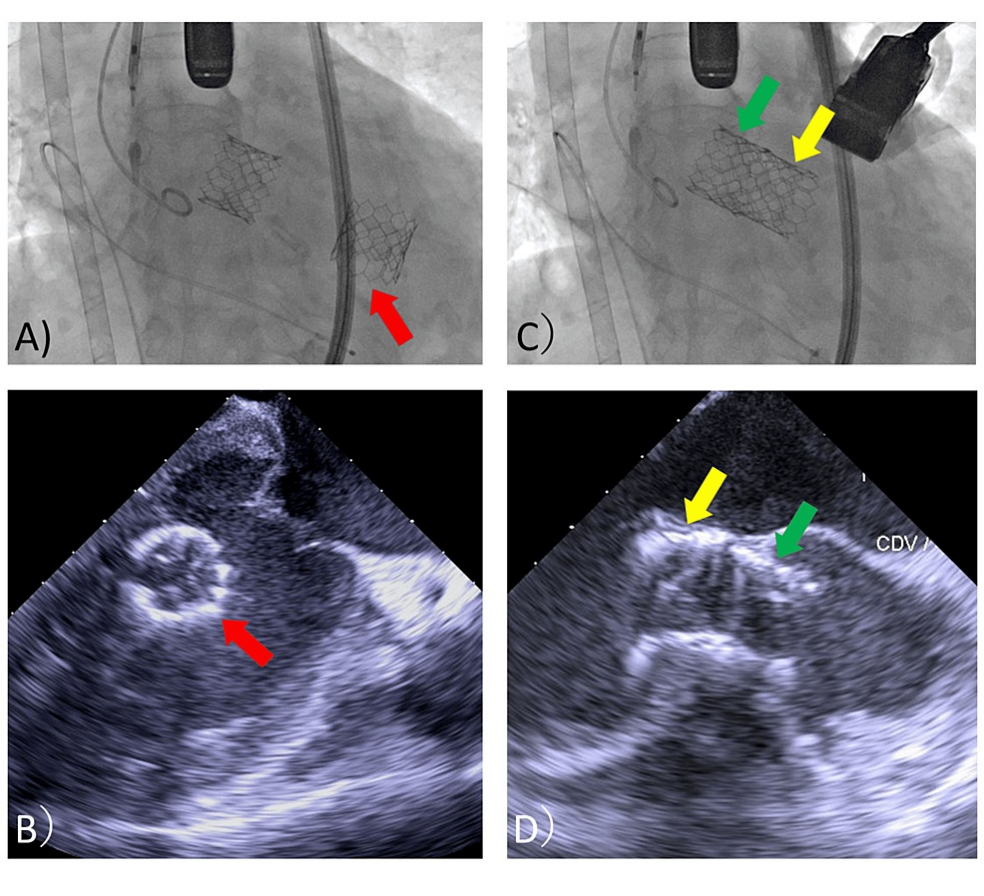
Fluoroscopic and TEE images of the migrated first TAVR valve A and B: A rotating TAVR valve is observed in the LV. Red arrows indicate the retrograde, migrated first valve floating in the LV. C and D: Autonomous insertion of the migrated TAVR valve in the LV outlet in reverse valve position. Yellow arrows indicate the first valve stuck in the LVOT in the reversed position. Green arrows show the second valve implanted in the aortic valve position. TEE: Transesophageal echocardiography, TAVR: Transcatheter aortic valve replacement, LV: Left ventricle, LVOT: Left ventricular outflow tract
